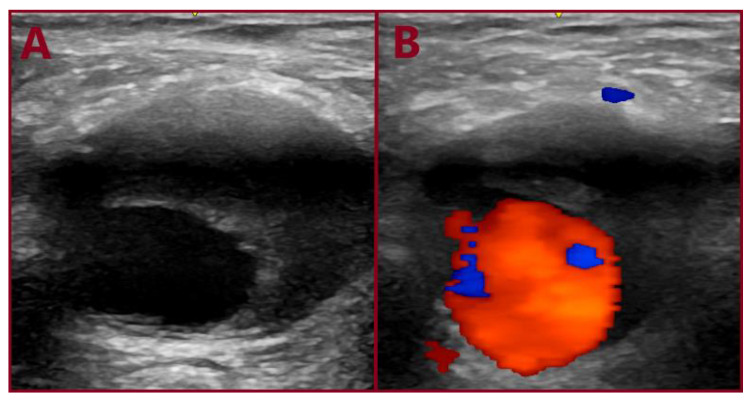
Ultrasonographic appearance of the common femoral artery aneurysm: (A) gray-scale; (B) color duplex imaging.
